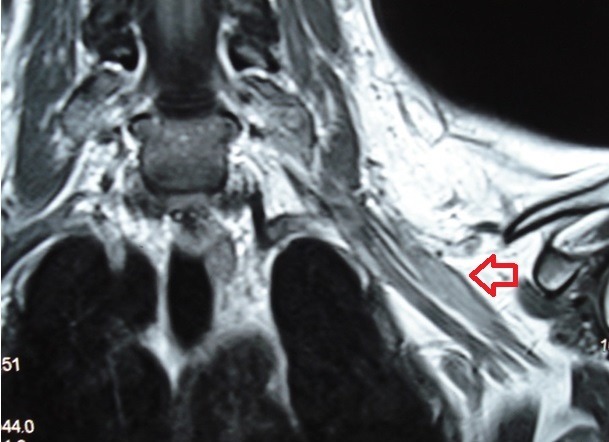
MRI showing typical aspect of hyperintense T2-weighted images on the posterior trunk of the left brachial plexus
